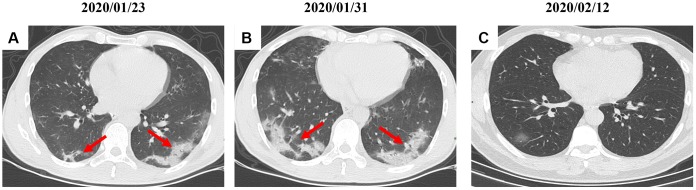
A serial CT images after admission of a 54-year-old female patient. (A) Patch ground-glass opacity mainly located in the left lower lobe. (B) Significant larger patch ground-glass opacities were observed in both lower lobes after 8 days. (C) Follow-up CT scans on day 20 after admission show a remarkable improvement. Typical lesions were marked with red arrows.
